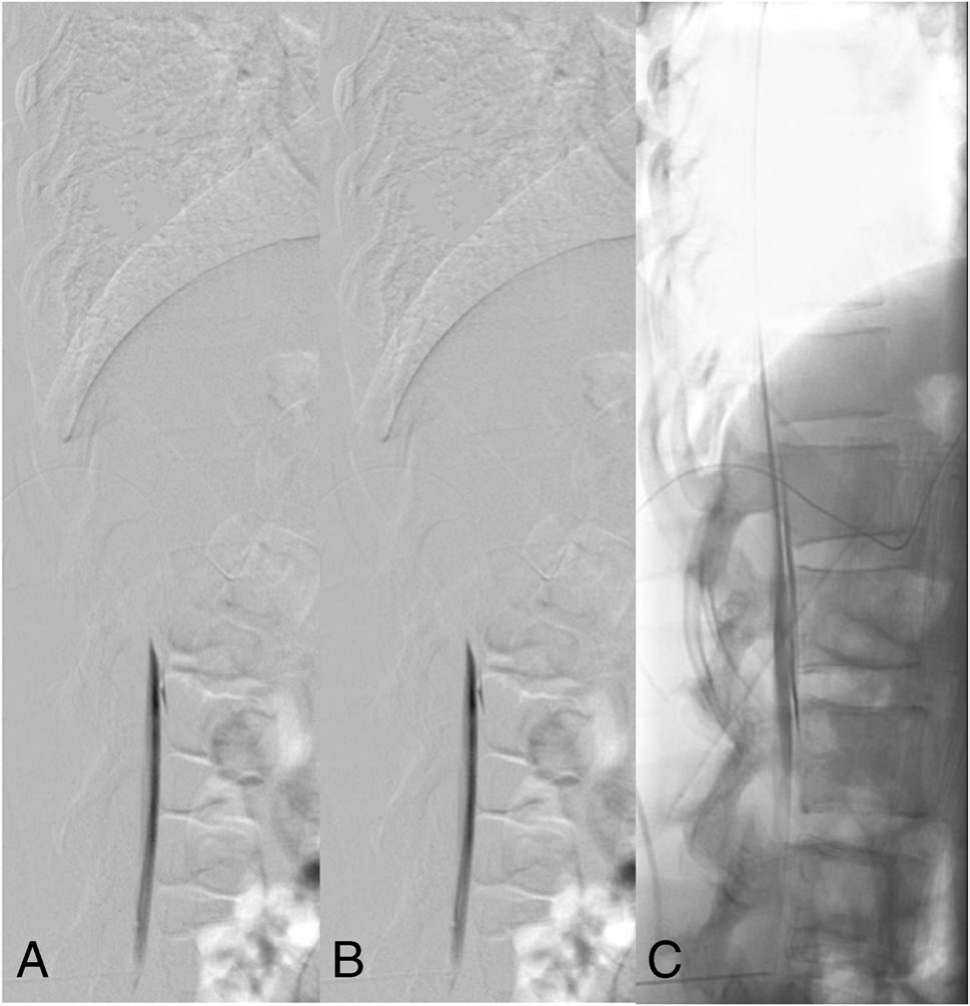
Type 1 leak: Dynamic digital subtraction myelogram (DSM) with the patient in prone position on a tilted table. Due to the higher specific weight compared to CSF, iodinated contrast medium collects at the ventral surface of the thecal sac. With the X-ray tube in lateral and the patient in head down position (around 15°), digital subtraction myelograms are acquired during gentle injection of highly concentrated (300 mg/ml) contrast medium. Using this technique, most of the ventral leaks in the lower thoracic and lumbar spine can be visualized. In this 30-year-old woman, dynamic DSM shows a ventral leak at the level L1/2 (A, B). Note that on the subsequent unsubtracted fluoroscopic image the exact site of the leak cannot be determined any longer (C)
